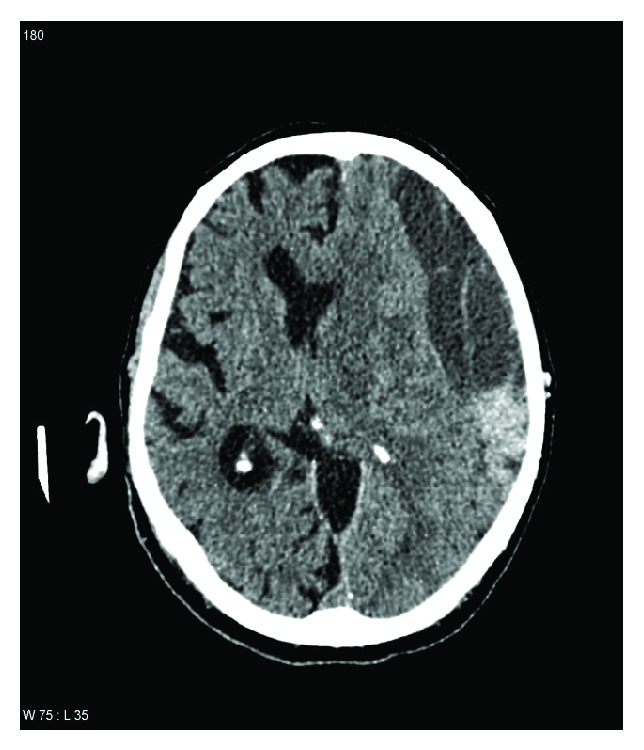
Computed tomography of a left-sided chronic subdural haematoma.
